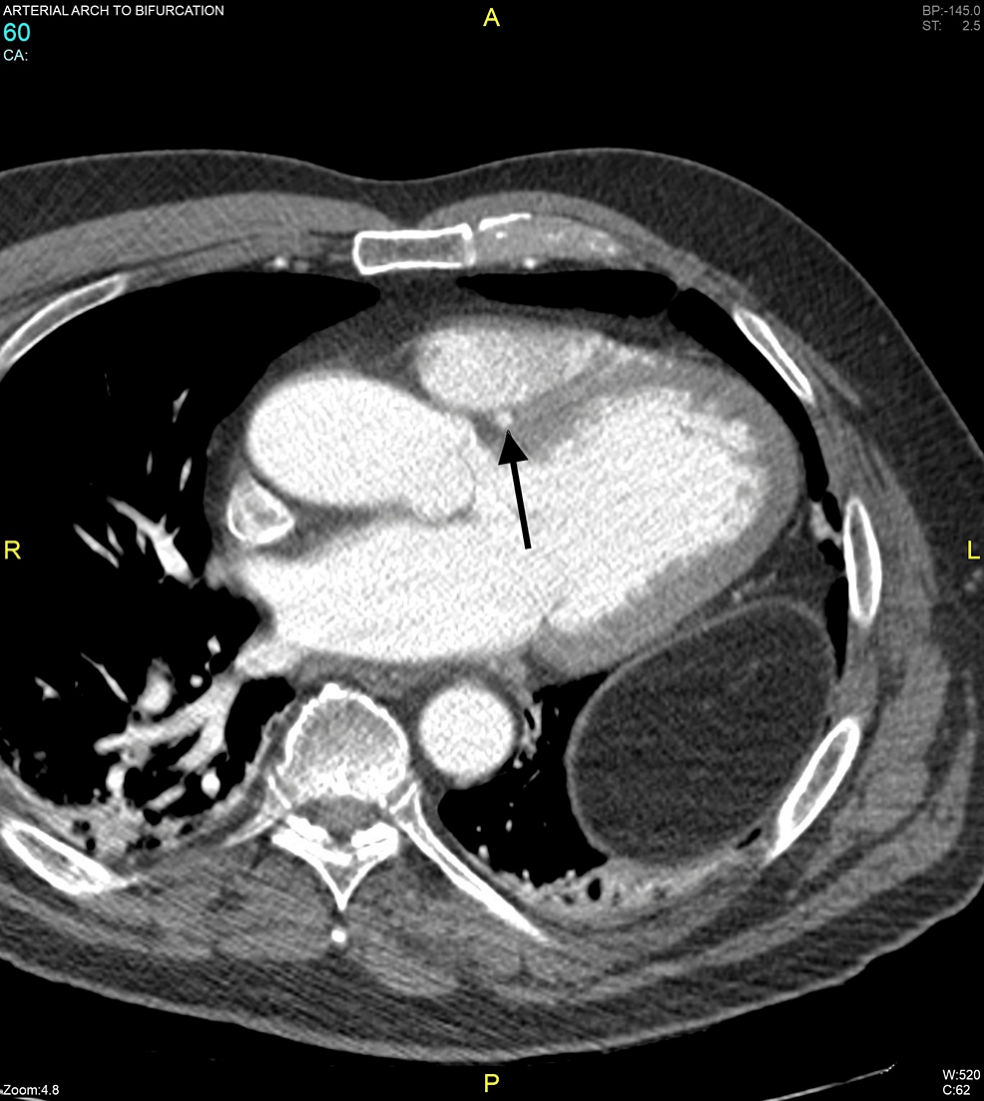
CT angiography (axial view) showing the septal course of the LMCA (black arrow) LMCA: left main coronary artery
